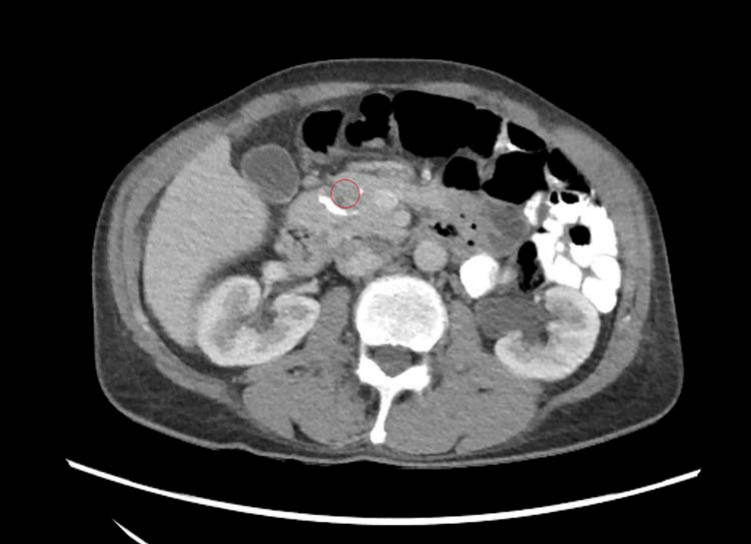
Contrast enhanced CT scan of pancreas.
